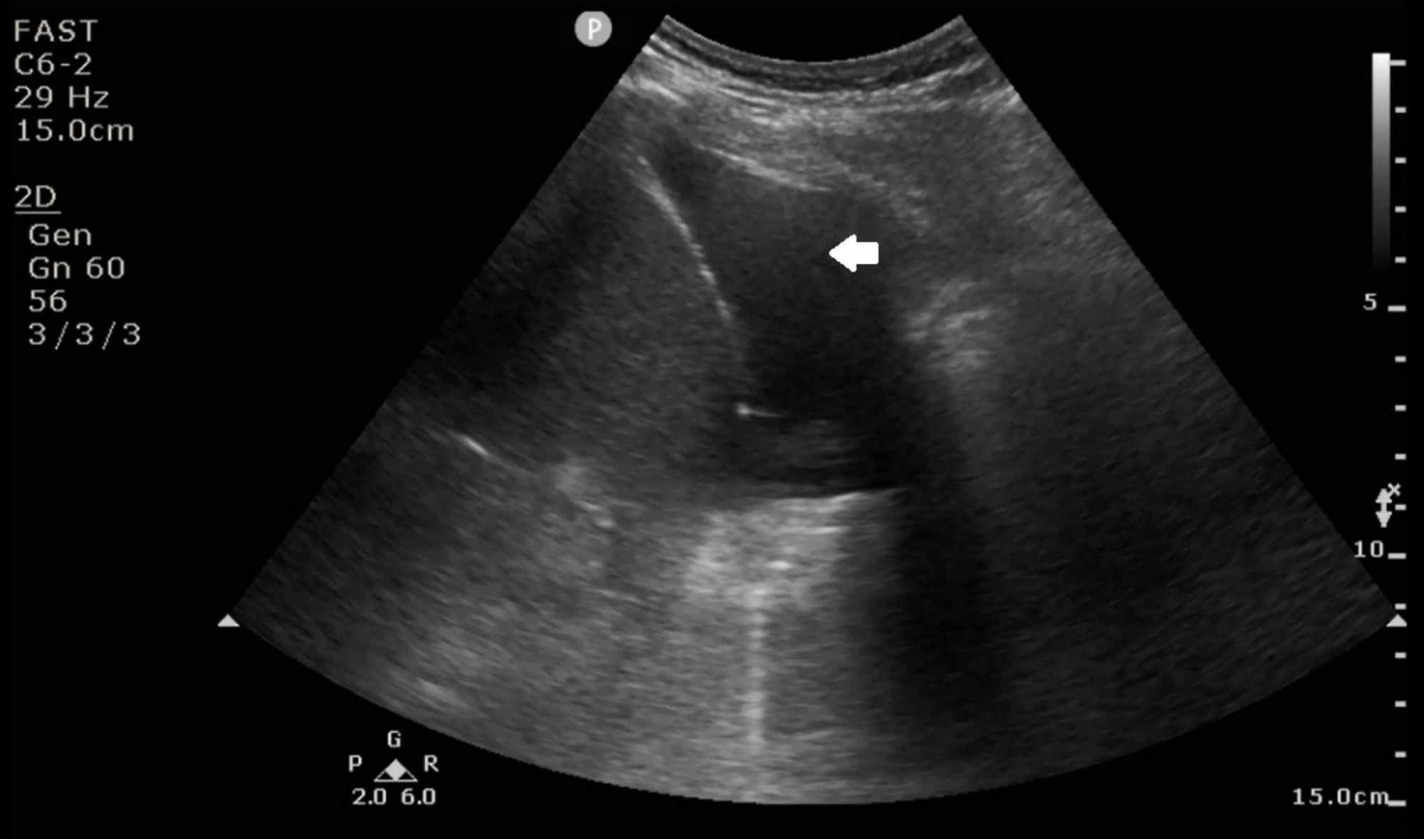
Ultrasound showing the moderate size right sided pleural effusion (arrow)
